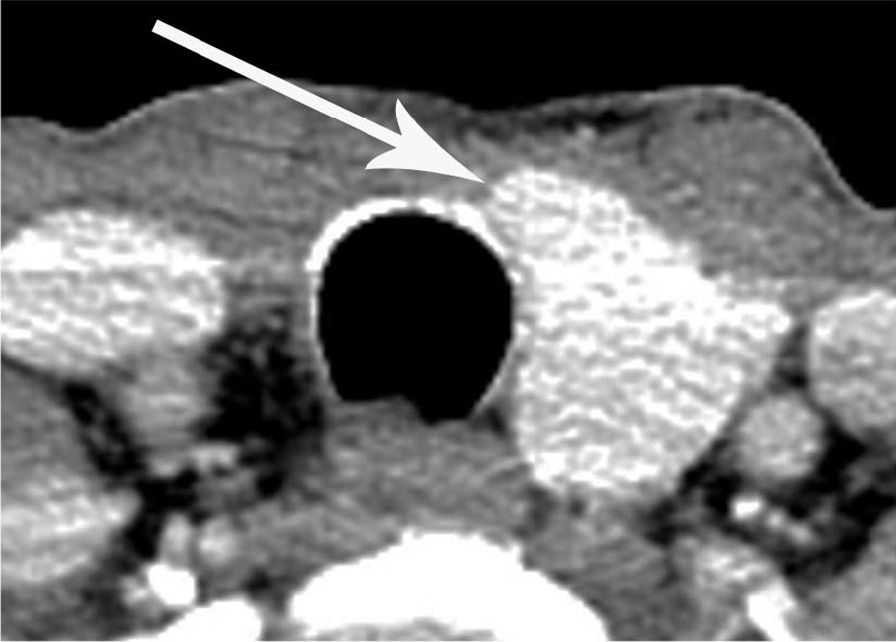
A 65-year-old man with right hemithyroidectomy for papillary thyroid cancer. Axial contrast-enhanced CT scan shows the absence of isthmus and blunt edge of medial end of remnant thyroid gland (arrow). The angle of the medial end of remnant thyroid gland was measured as + 24°
